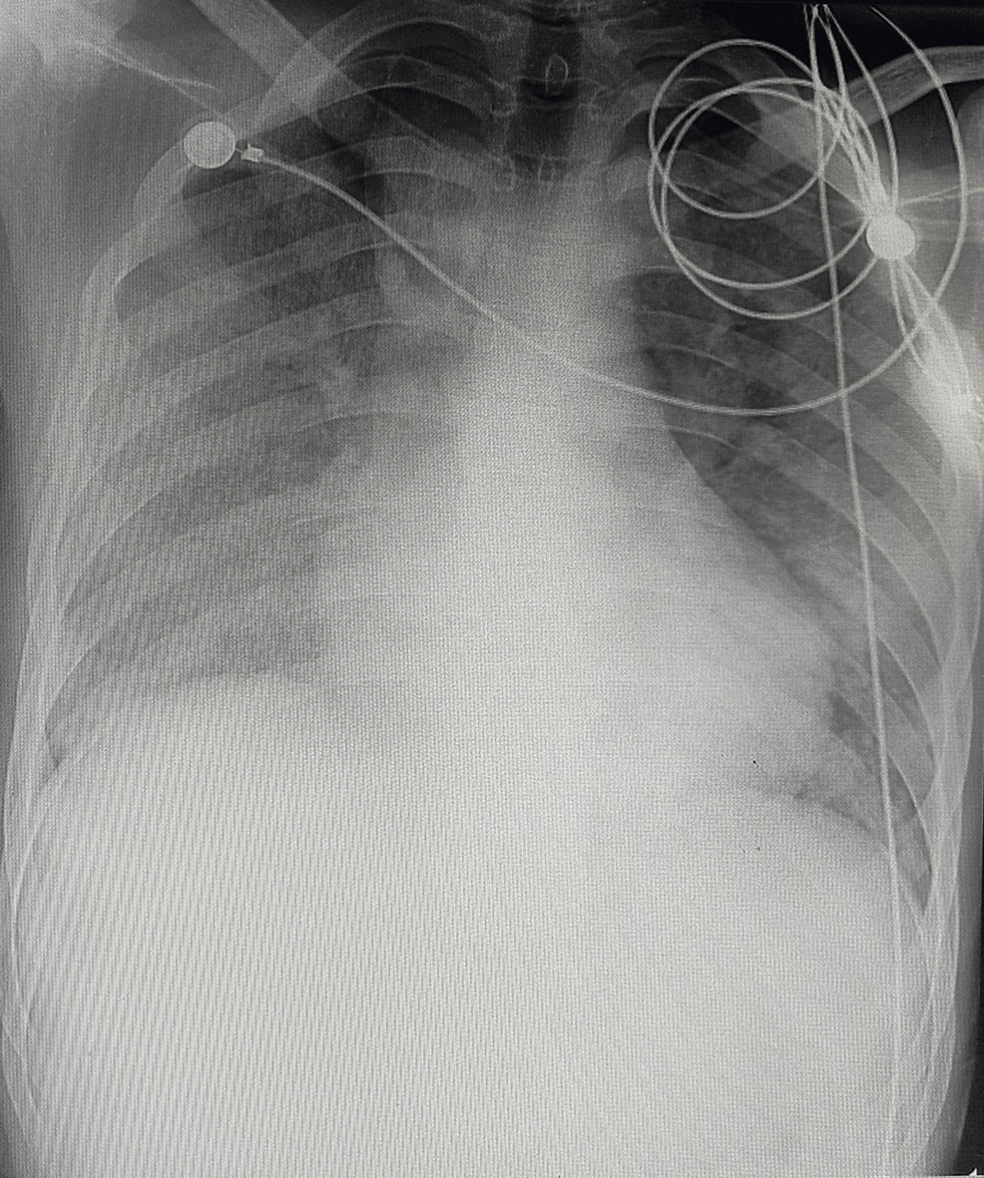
Chest X-ray showing improvement after ICU management.
